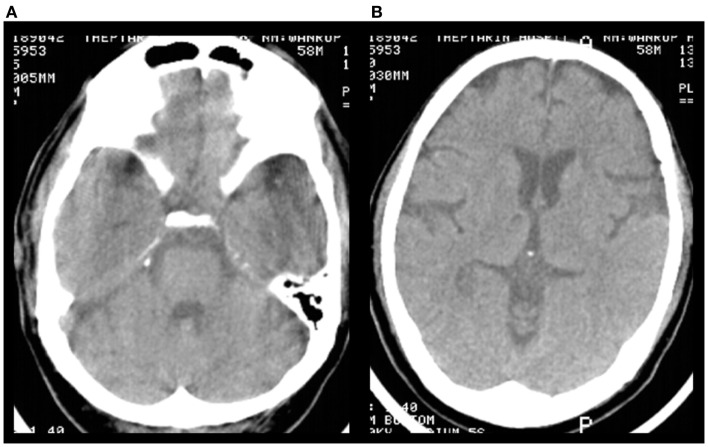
Pre-treatment non-contrast brain CT scan. (A,B) There was no evidence of acute ischemic or hemorrhagic stroke.
